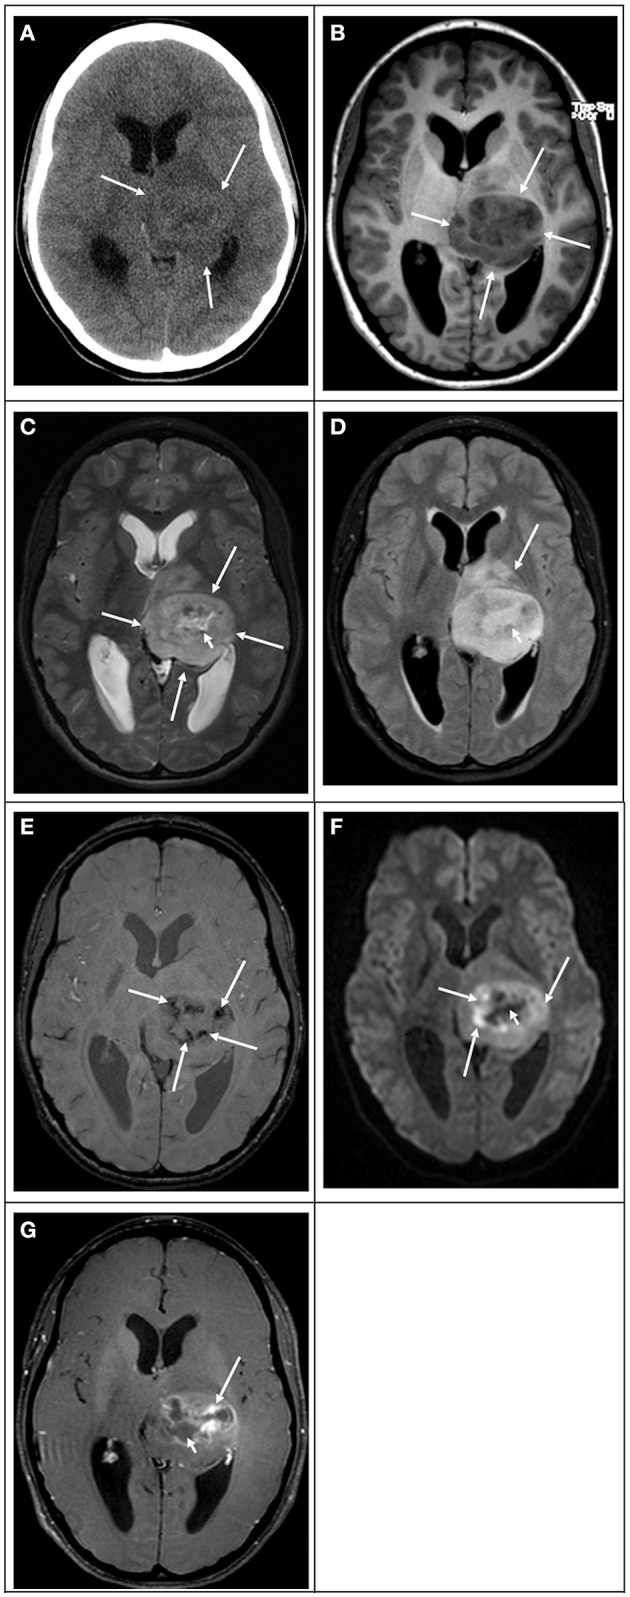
13-year-old female with blurry vision, facial weakness, and right-sided paresthesias due to a left thalamic glioblastoma. (A) Computed tomography image in the axial plane shows an ill-defined slightly hyperdense mass in the left thalamus. (B) T1 weighted image in the axial plane shows that the lesion is well-demarcated with heterogeneous mixed-signal, predominantly hypointense (white arrows). (C) T2 weighted image and (D) FLAIR images in the axial plane show that the bulk of the lesion is hyperintense (white arrows) with a central component showing even higher signal. (E) Susceptibility weighted image in the axial plane shows irregular signal drop foci within the tumoral bed (white arrows) in keeping with hemorrhage. (F) Diffusion-weighted image in the axial plane shows that the lesion is heterogeneous in signal. The periphery of the mass is isointense to the normal brain parenchyma. The center of the mass shows a hypointense signal (white arrow). Surrounding the center, there is an irregular hyperintense thick rim with low values in the ADC map (not shown) in keeping with restricted diffusion. (G) T1-weighted contrast-enhanced image in the axial plane shows that the tumor enhances heterogeneously. The thick irregular area that shows restricted diffusion enhances avidly (white arrows) with no enhancement centrally due to necrosis.
